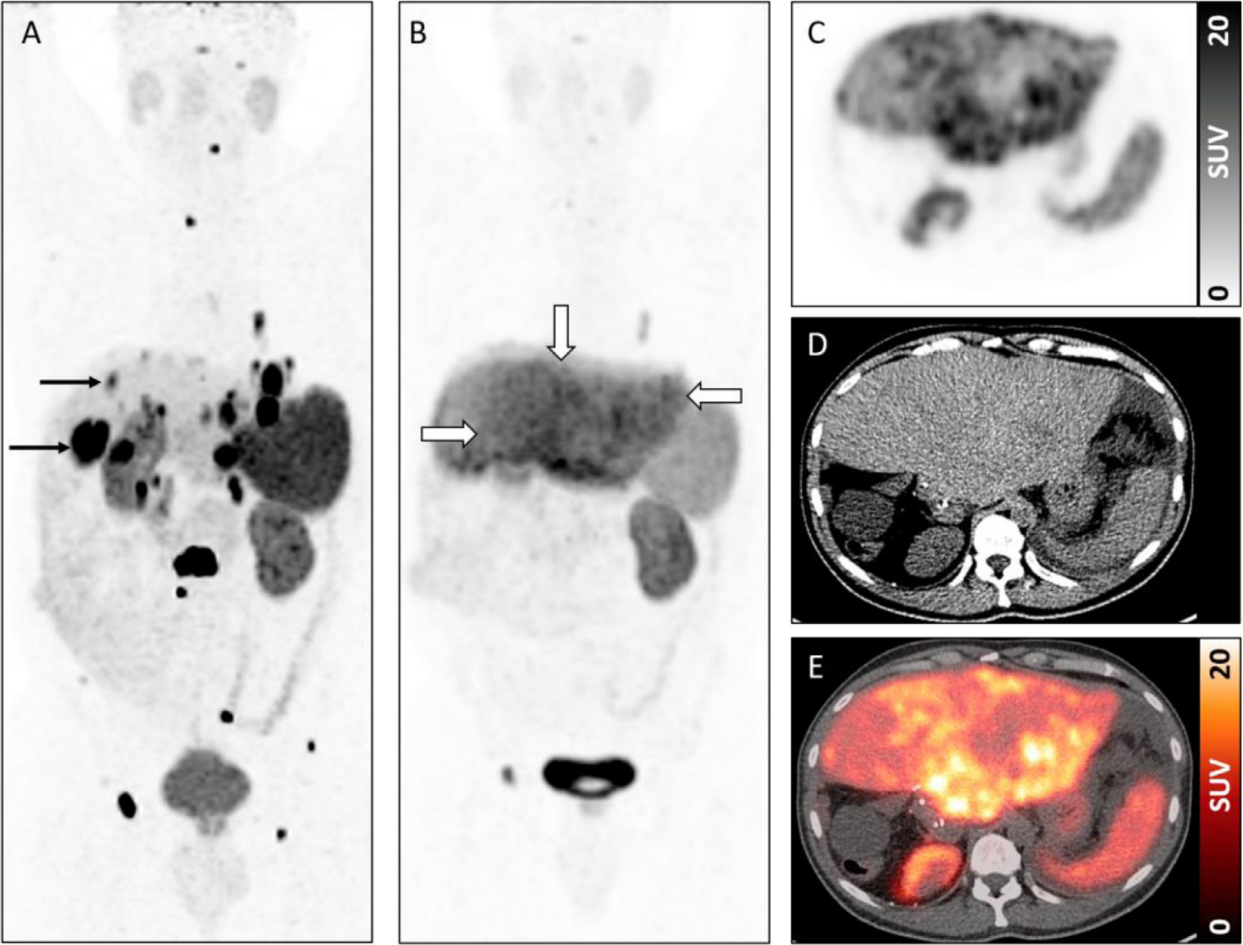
[^68^Ga]DOTATATE PET/CT from 3 years prior (A) and at the current time (B-E). Current [^68^Ga]DOTATATE PET/CT demonstrates resolution of discrete hepatic metastases seen on prior study (A), but appearance of diffusely increased uptake throughout the liver (B-E).
